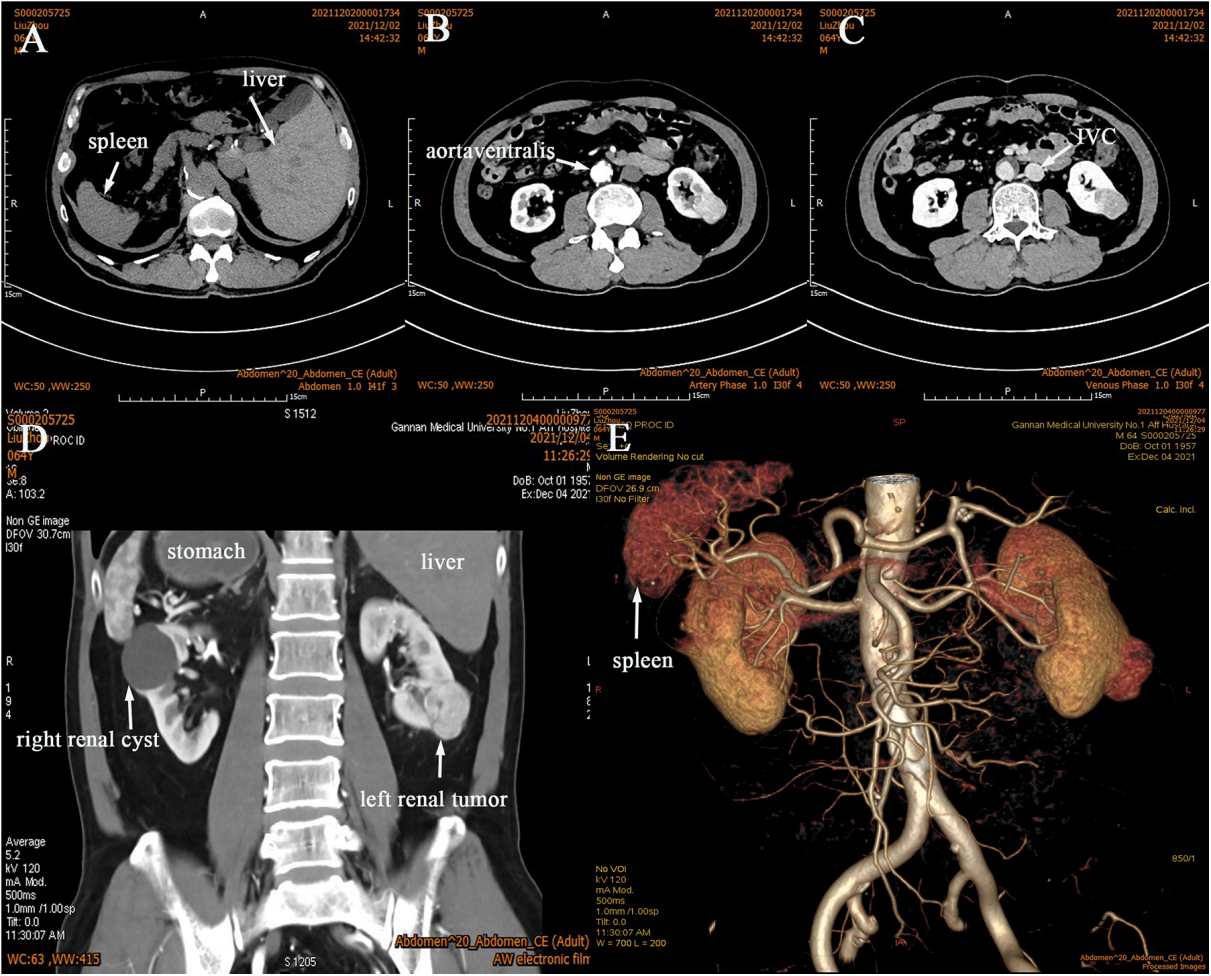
CTU and CTA was performed, and it confirmed SIT (A–E) with a tumor in the left kidney (B–E). It showed that the left renal artery had anatomical variation (E), the arterial phase (B), the venous phase (C), the coronal view (D).
